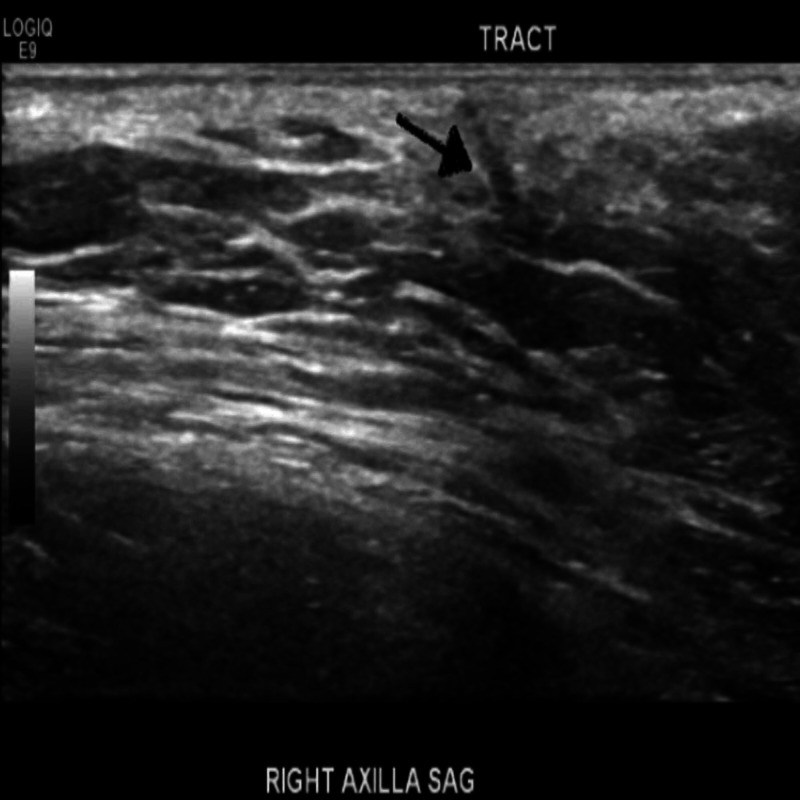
Right axillary ultrasound showing a vague hypoechoic area with an apparent tract extending through the skin (black arrow pointing the tract).
